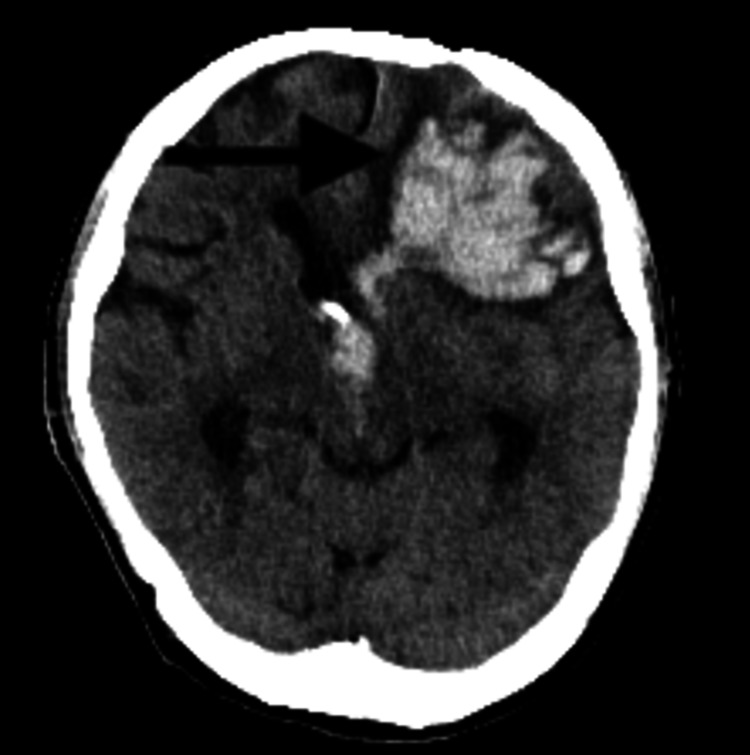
CT of the head/brain without contrast showing a large left frontal intraparenchymal hematoma measuring up to 5.7cm with surrounding edema and intraventricular hemorrhage within the lateral, third, and fourth ventricles. CT, computed tomography.
